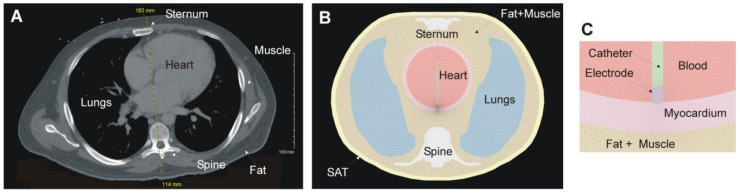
(A) CT-scan slice from a representative patient. (B) Computer model (units in mm) of posterior RFCA inspired by the CT-scan image and including the most representative organs. (C) Zoom of the RF catheter and cardiac wall.
